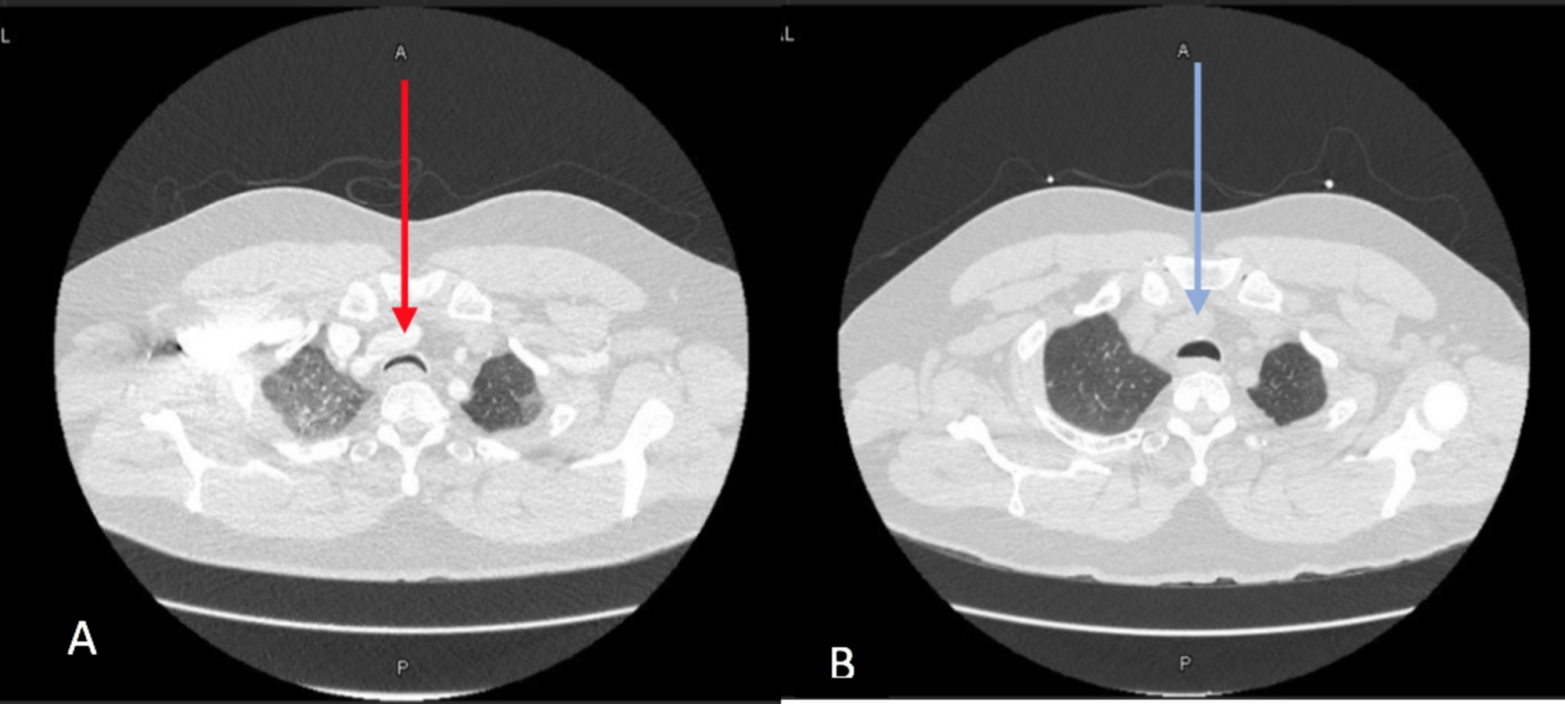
CT chest axial images showing A) severe TM (red arrow) in proximal airway along with diffuse GGO bilaterally B) showing improvement in TM (blue arrow) and GGO. TM, tracheomalacia; GGO, ground glass opacities
